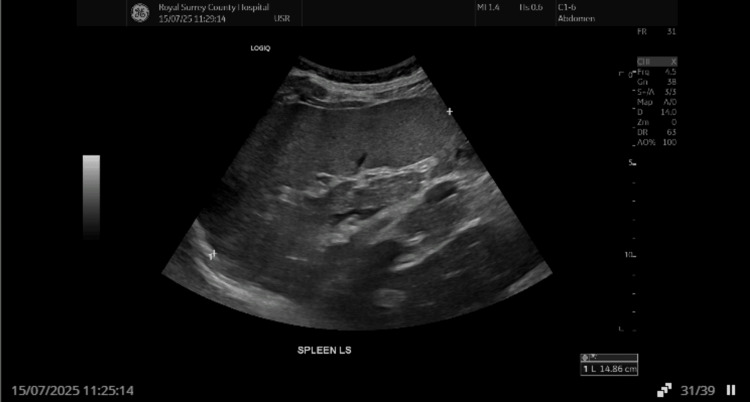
Follow-up abdominal ultrasound Four months post-presentation, ultrasound imaging demonstrating complete resolution of the splenic infarct. The spleen now measures 9.38 cm with normal homogeneous echotexture and no focal lesion visible.
